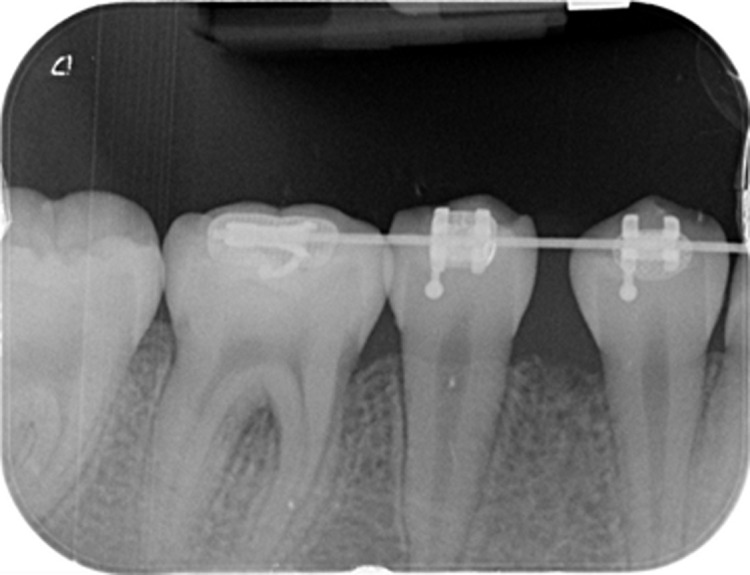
retroalveolar X-ray of teeth 44 to 47
